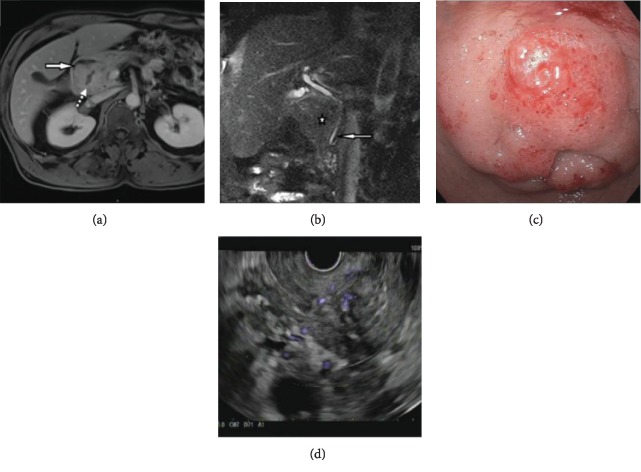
Preoperative endoscopy and imaging. (a) T1 VIBE postcontrast sequence axial MRI acquisition showing a delayed enhancement of the 4.4-centimeter mass along the medial duodenal wall with few internal cystic areas (dashed arrow) and accumulation of fluid in the right anterior paraduodenal space (arrow). (b) T2 HASTE sequence coronal MRI acquisition showing the mass along the duodenum (star) and the distal part of the common bile duct winding around the mass with narrowing at the tip (arrow). (c) Endoscopic appearance of the duodenum showing a polypoid and eroded mucosa. (d) EUS showing an ill-defined heterogeneous paraduodenal lesion causing distortion of the duodenal wall layers.
